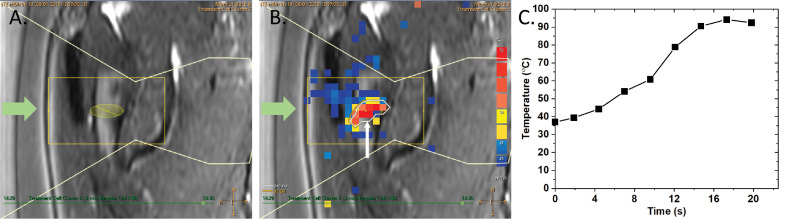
Representative example of a MR-HIFU ablation using a 4 mm treatment cell, 1.2 MHz, 60 W sonication power and 14.9 s sonication duration. The green arrows (A, B) depict the direction of incoming ultrasound energy. (A) A treatment cell (ellipsoid) positioned on the desmoid tumor. (B) Temperature map of a sonication. White contour and arrow mark the 240CEM43 thermal dose. (C) The corresponding temperature increase over time within the treatment cell during a sonication.
